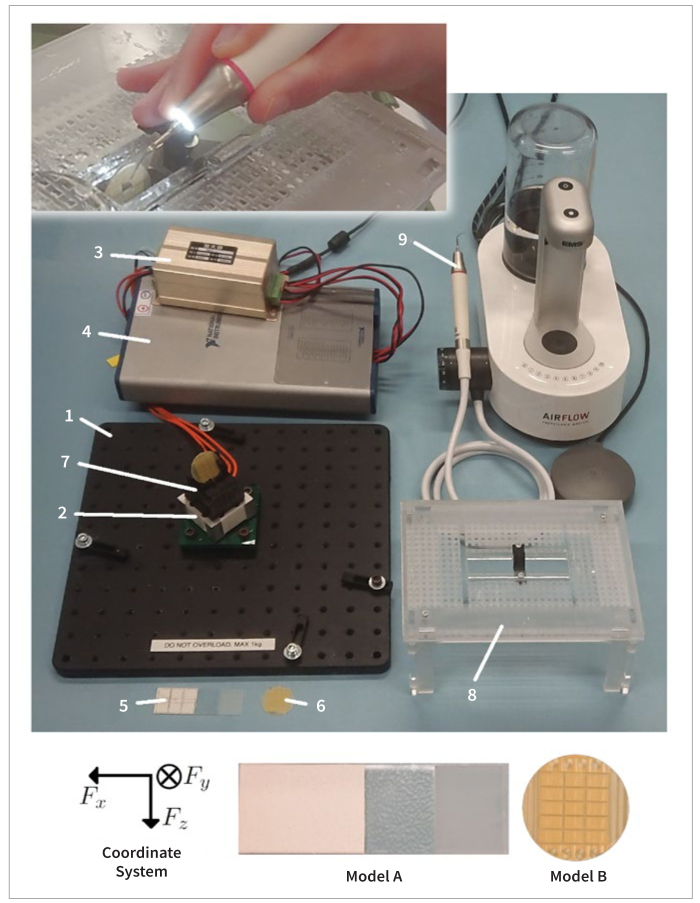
Image taken during debridement (top left) using the measurement set-up (center): base plate (1); three-axis force sensor (2) with amplifier (3); data acquisition module (4); Model A (5) with comparatively soft and Model B (6) with comparatively hard artificial dental calculus; adapters (7), handrest (8); and piezoelectric ultrasonic scaler (9) and definition of coordinate system aligned to Model A and Model B (bottom).
